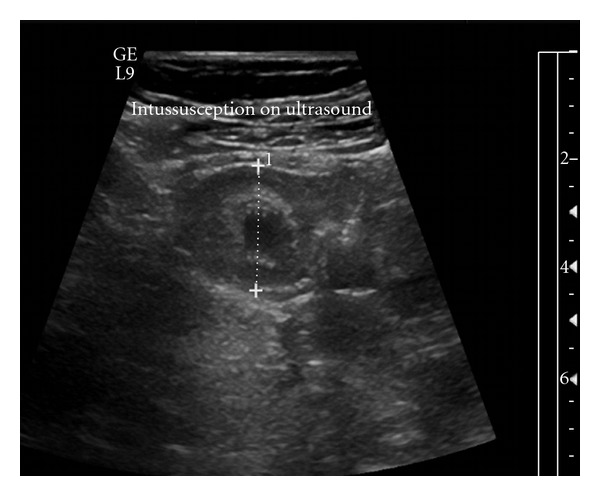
Transabdominal ultrasound with mass in right lower quadrant.
